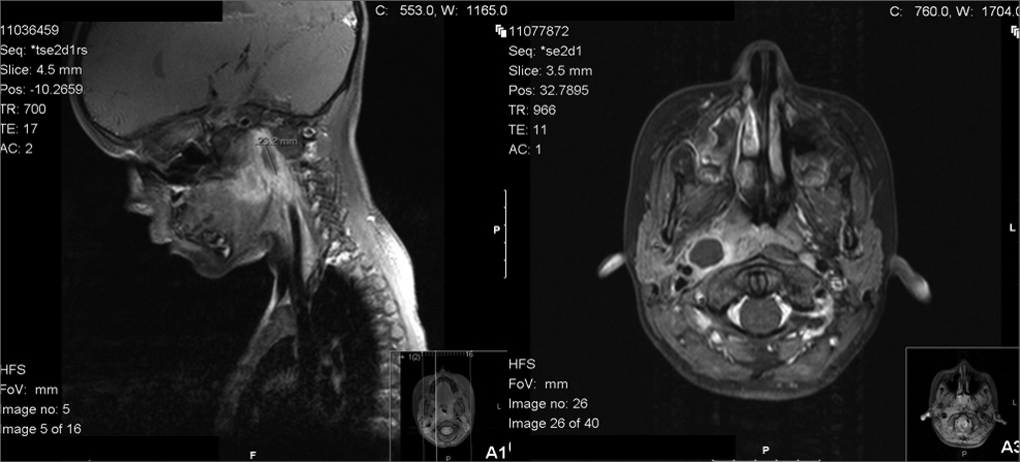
A. Lateropharyngeal abscess (MRI scan, sagittal view). B. Lateropharyngeal abscess (MRI scan, axial view)
